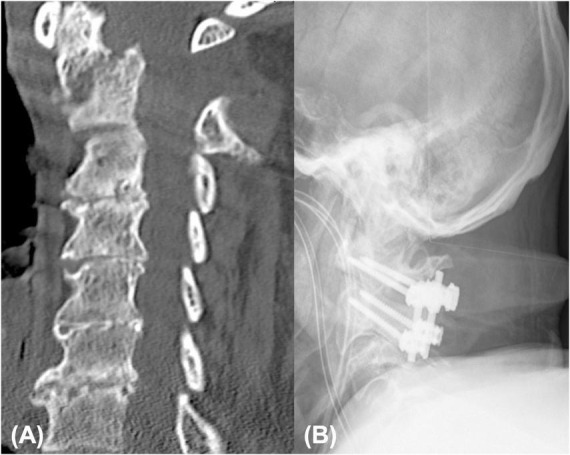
A total of 85 year male patient presenting with arm monoparesis: emergency CT showing type II odontoid fracture with anterior displacement (A), post-operative anterior-posterior radiographs displaying posterior screw fixation of C1/C2 (B).
